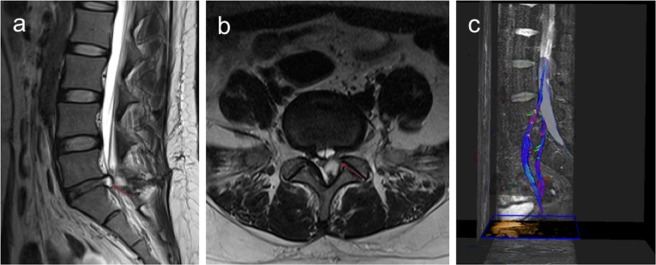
Lumbar disc MRI and lumbosacral nerve root DTI scan at 30 days post-surgery. (a) is the sagittal position of the patient’s MRI 30 days post-surgery, showing an obvious “grape skin” phenomenon. (b) is the patient’s MRI axial position 30 days post-surgery, showing the spinal canal is not compressed. (c) is the nerve root reconstructed by DTI 30 days post-surgery, partial recovery of the compressed nerve root microstructure was observed.
